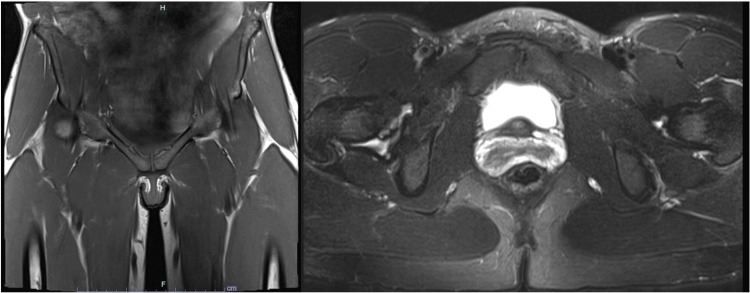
MRI from eight-month follow-up This figure shows MRI results of the patient, performed approximately eight months after the initial robotic-assisted inguinal hernia repair. Note that there appears to be no evidence of inflammation near the round ligament insertion and the mons pubis, or of hernia recurrence. The patient was not currently experiencing symptoms at the time of this study. MRI, magnetic resonance imaging.
